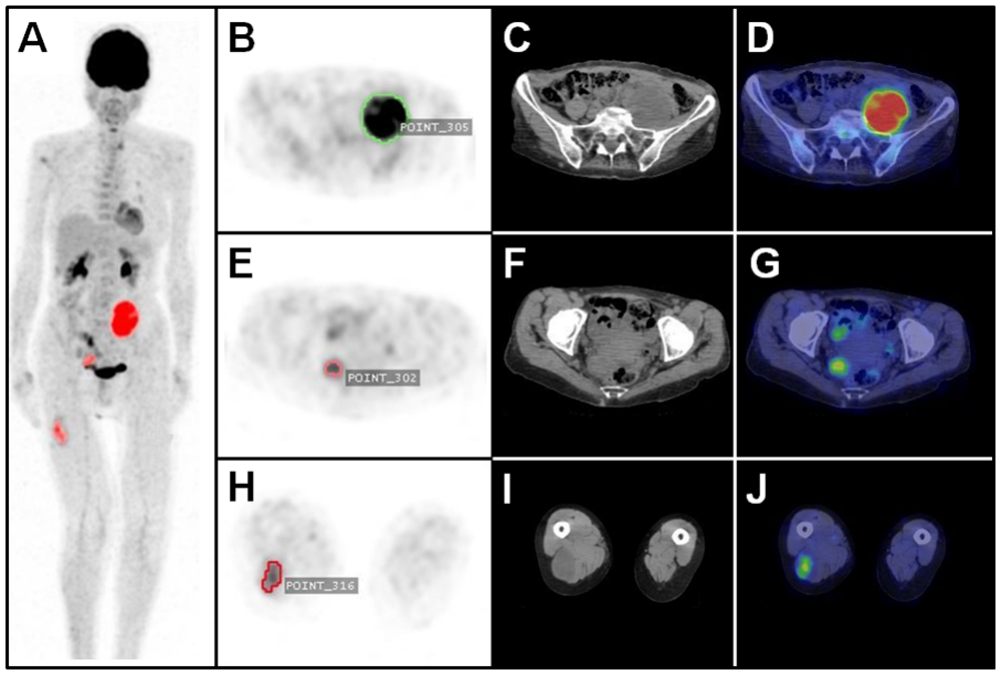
Automated 3D-segmentation and volumetric assessment of nerve sheath tumors. Maximum intensity projection PET image (A) with automatically delineated metabolically active tumors (*red*). Axial PET image (B) with volumetric ROI (*green*) of a MPNST (SUV_max_ / MTV / TLG: 11.2 / 182592 / 894700) in the left pelvis; corresponding CT (C) and fused PET/CT image (D). Axial PET image (E) with volumetric ROI (*light red*) of a plexiform neurofibroma (PNF) (SUV_max_ / MTV / TLG: 4.7 / 14848 / 41574) close to the rectum; corresponding CT (F) and PET/CT image (G). Axial PET image (H) with volumetric ROI (*dark red*) of a BPNST (SUV_max_ / MTV / TLG: 3.7 / 15872 / 41267) in the right thigh; corresponding CT (I) and PET/CT image (J).
